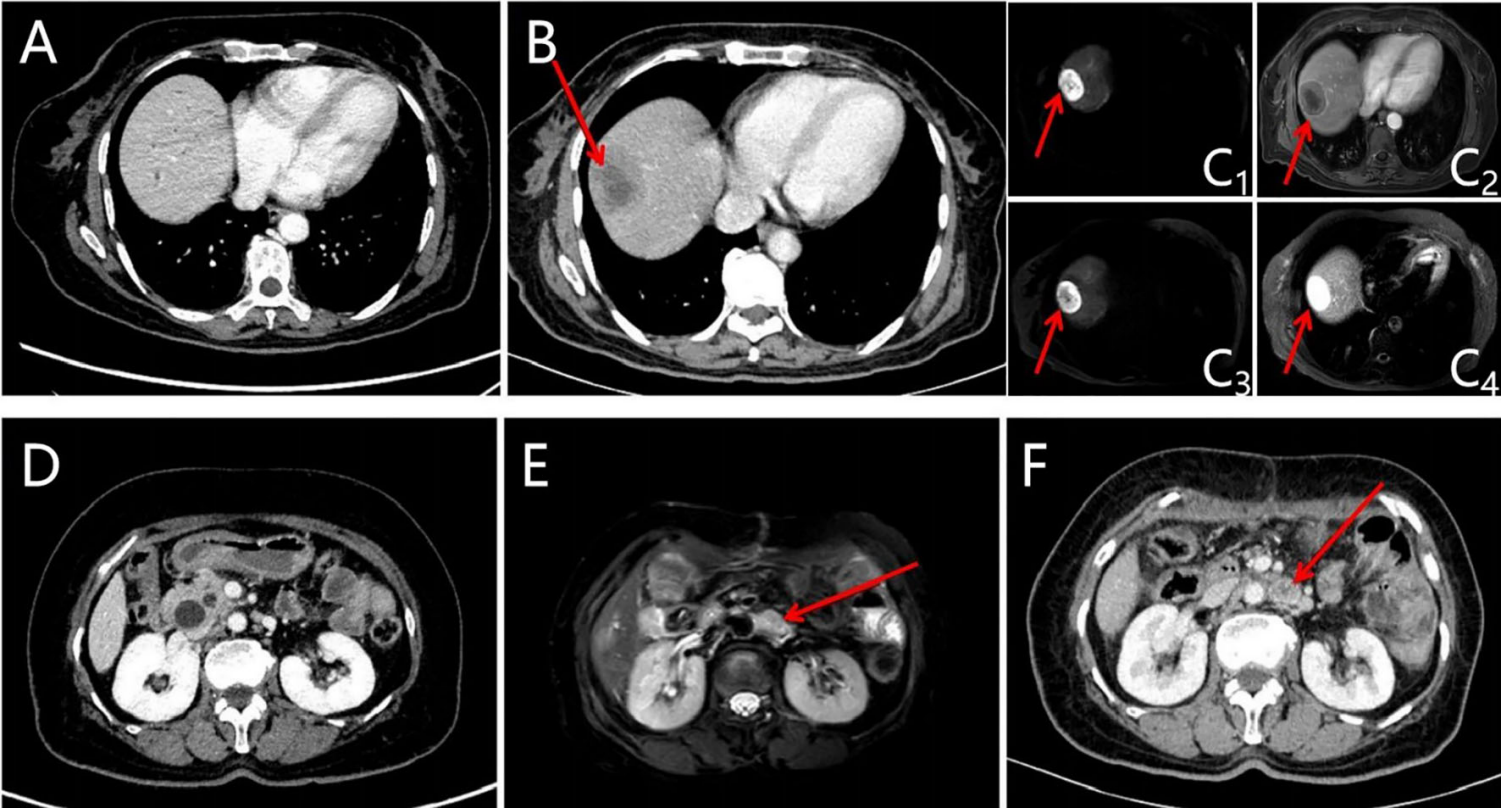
Images of the liver and retroperitoneal lymph node lesions were evaluated before and after the operation (new liver and retroperitoneal lymph node lesions after operation, shown by red arrows). (A) CT of liver before the operation; (B) CT of liver was evaluated at baseline after the operation; (C) MRI of the liver was evaluated at baseline after the operation (C_1_: DWI-b50; C_2_: T1WI; C_3_: DWI-b800; C_4_: T2WI); (D) CT of retroperitoneal lymph nodes before the operation; (E) CT of retroperitoneal lymph nodes was evaluated at baseline after the operation; (F) MRI of retroperitoneal lymph nodes was evaluated at baseline after the operation.
